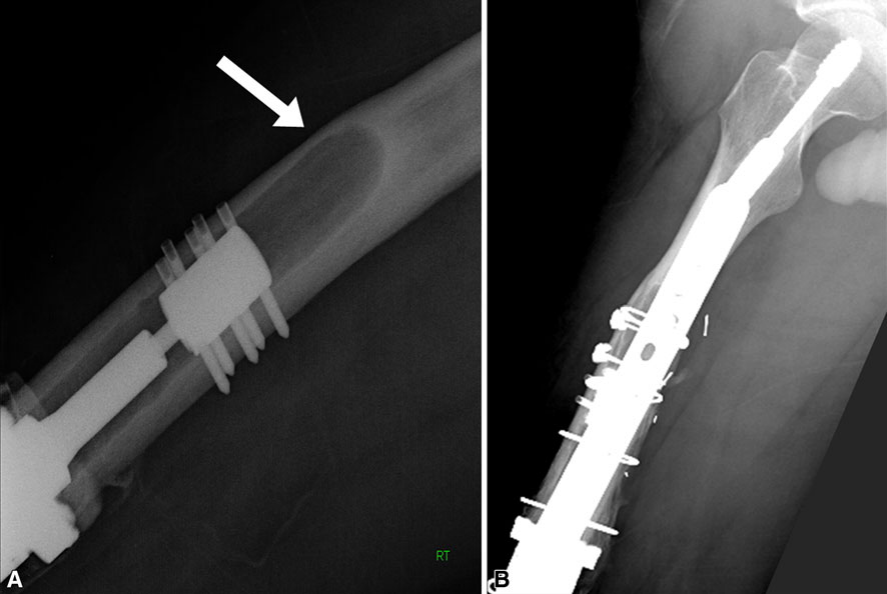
Type IIA failures are fractures proximal to the anchor plug fixation, perhaps at a stress transition point or, as in (A) this case where the bone was thin due to endosteal erosion from a failed prior stemmed implant (arrow). (B) The followup radiograph shows the fracture healed with a dynamic hip screw fixation and onlay allograft struts.
